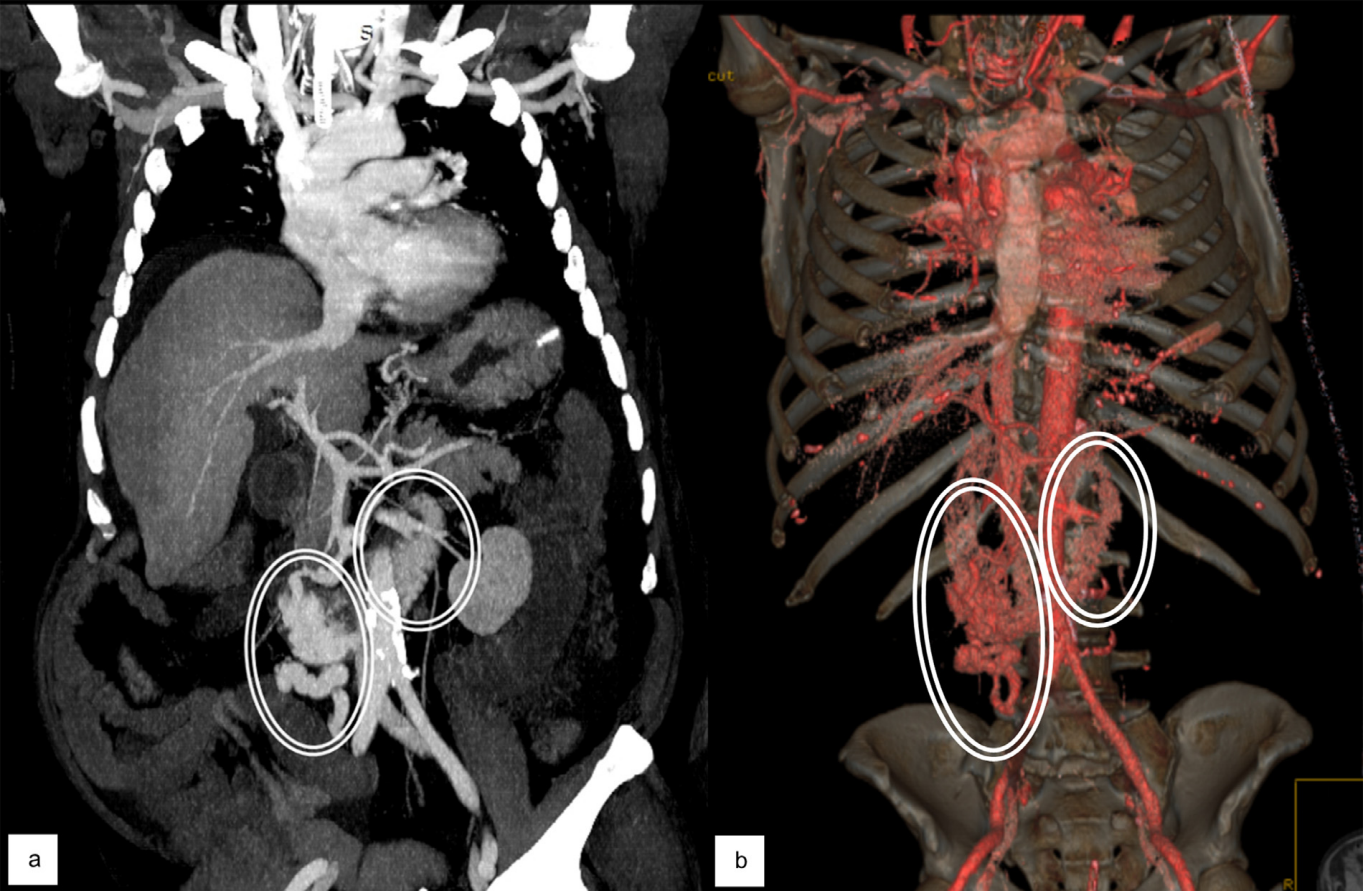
Coronal maximum intensity projection (MIP) (a) and 3d reconstruction (b) from contrast-enhanced CT demonstrating the presence of tortuous enlarged varicose vein with intraluminal duodenal and jejunal enhanced material due to active bleeding (circles).
